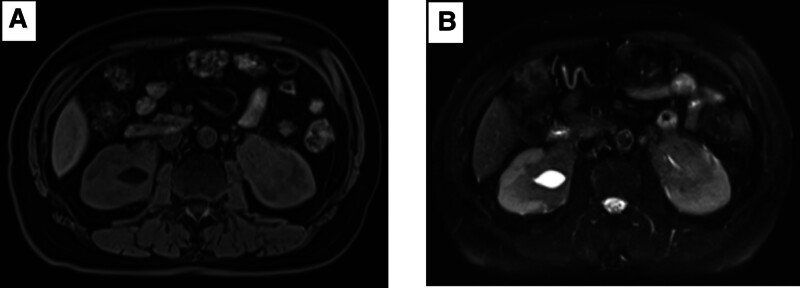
Abdominal MRI imaging showed space-occupying lesions at both renal pelvis.
